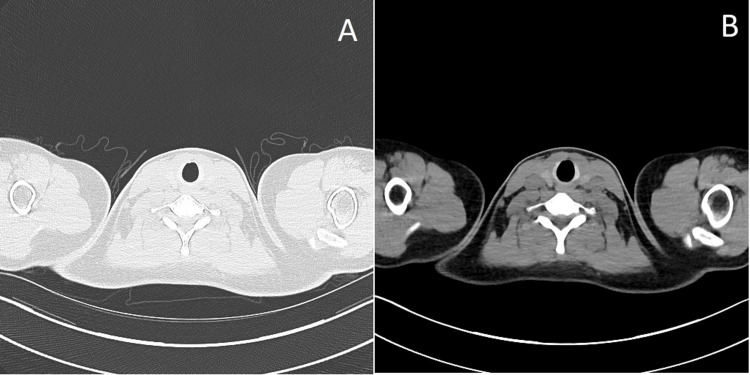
Control CT scan showing no remaining subcutaneous air (A) Thoracal imaging in lung window and (B) thoracal imaging in soft tissue window CT: computed tomography
